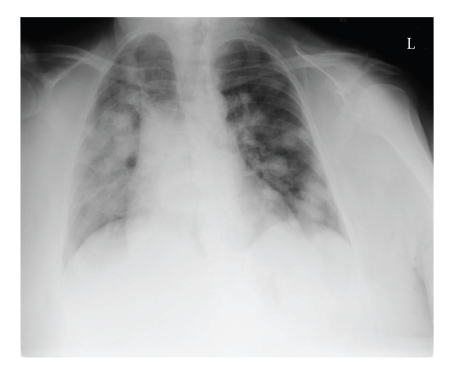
Chest X-ray showing multiple pulmonary nodules.
